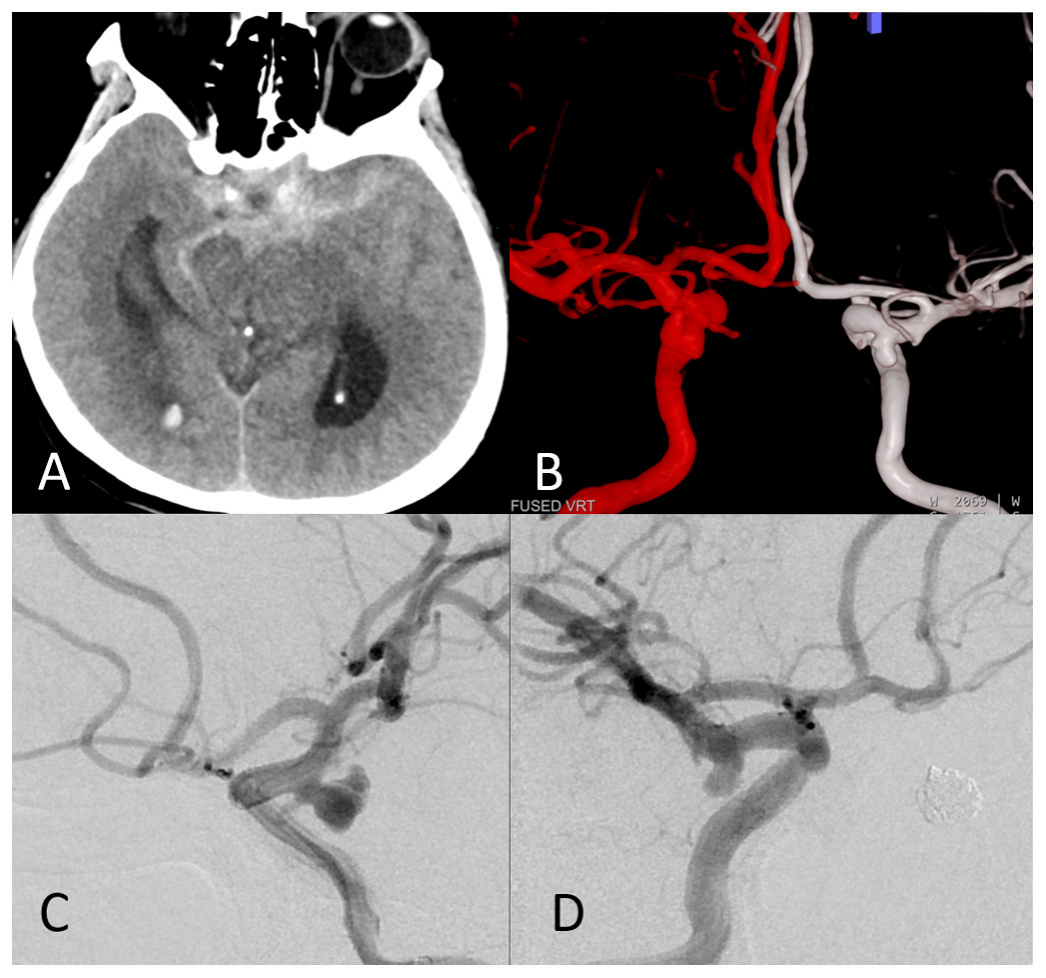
The images of a representative ruptured mirror posterior communicating artery aneurysm (PComAA). A 67-year-old woman presented with a severe headache. (A) Brain computed tomography (CT) scan showed subarachnoid hemorrhage (SAH) more on the left side. (B) Dual fusion of three-dimensional-digital subtraction angiography (3D-DSA) revealed a mirror PComAA. The left side of the mirror PComAA was more irregular than the right side. (C) The left internal carotid artery angiography revealed an irregular posterior communicating artery aneurysm that was a ruptured aneurysm. (D) The right internal carotid artery angiography showed a regular posterior communicating artery aneurysm.
